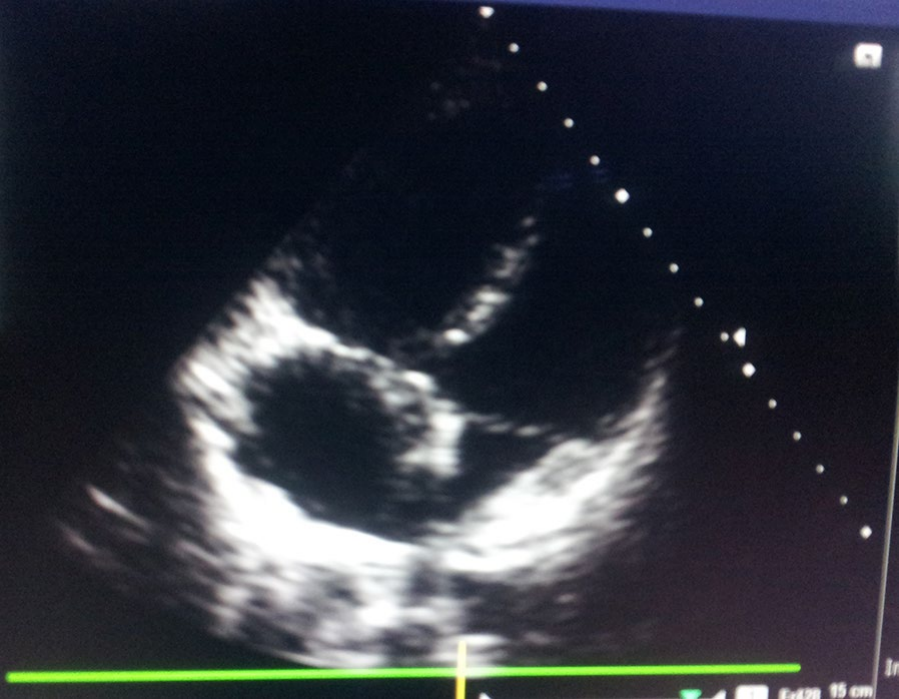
Trans-thoracic echocardiogram showing dilated right heart. Two-dimensional echocardiography in apical four chamber view showing dilated right atrium and right ventricle
